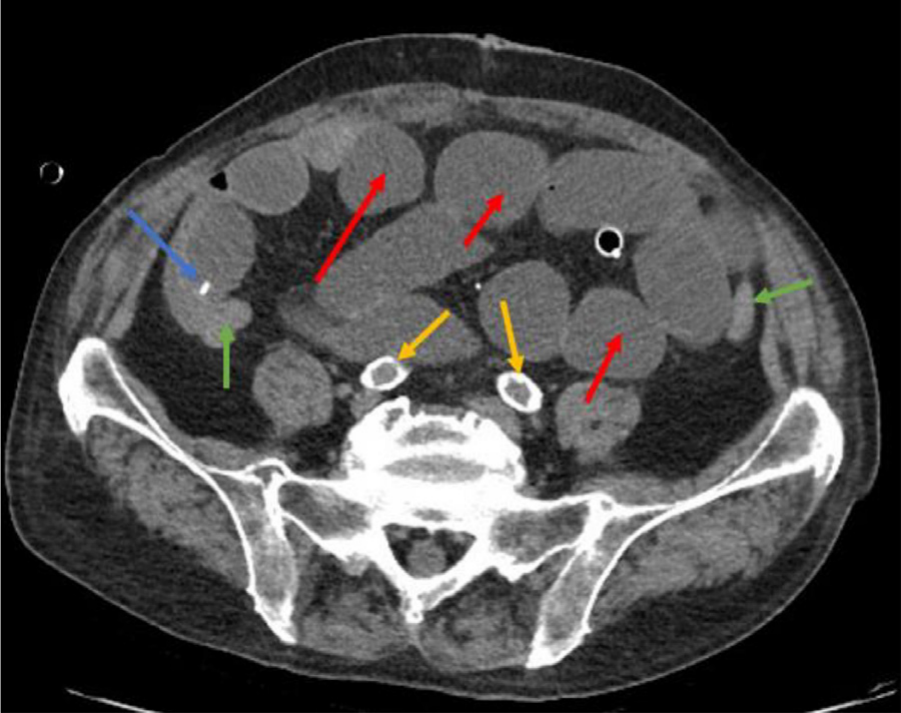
Noncontrast phase abdominal CT showing clips at the ileocecal valve (blue arrow), collapsed colonic walls (green arrows), and fluid-filled dilated ileal loops (red arrows) and diffuse calcified atherosclerosis involving the iliac arteries (yellow arrows).
